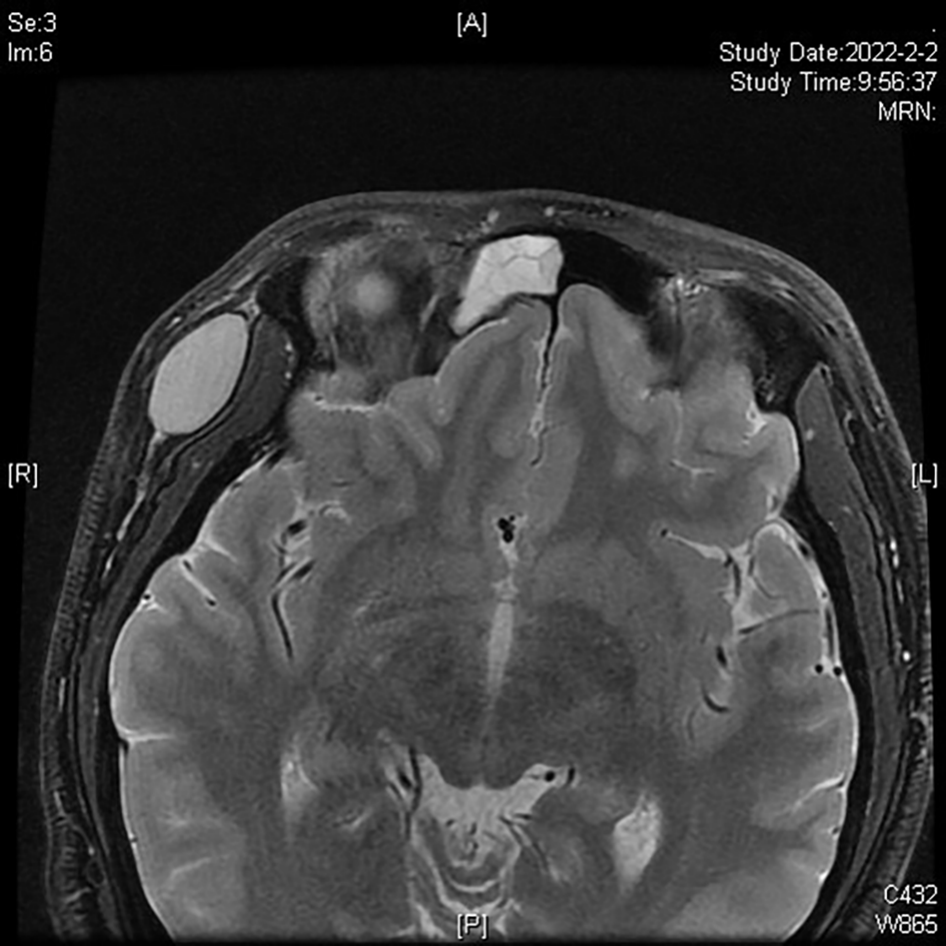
Orbital MRI showing a tumor in the right temporal area.
